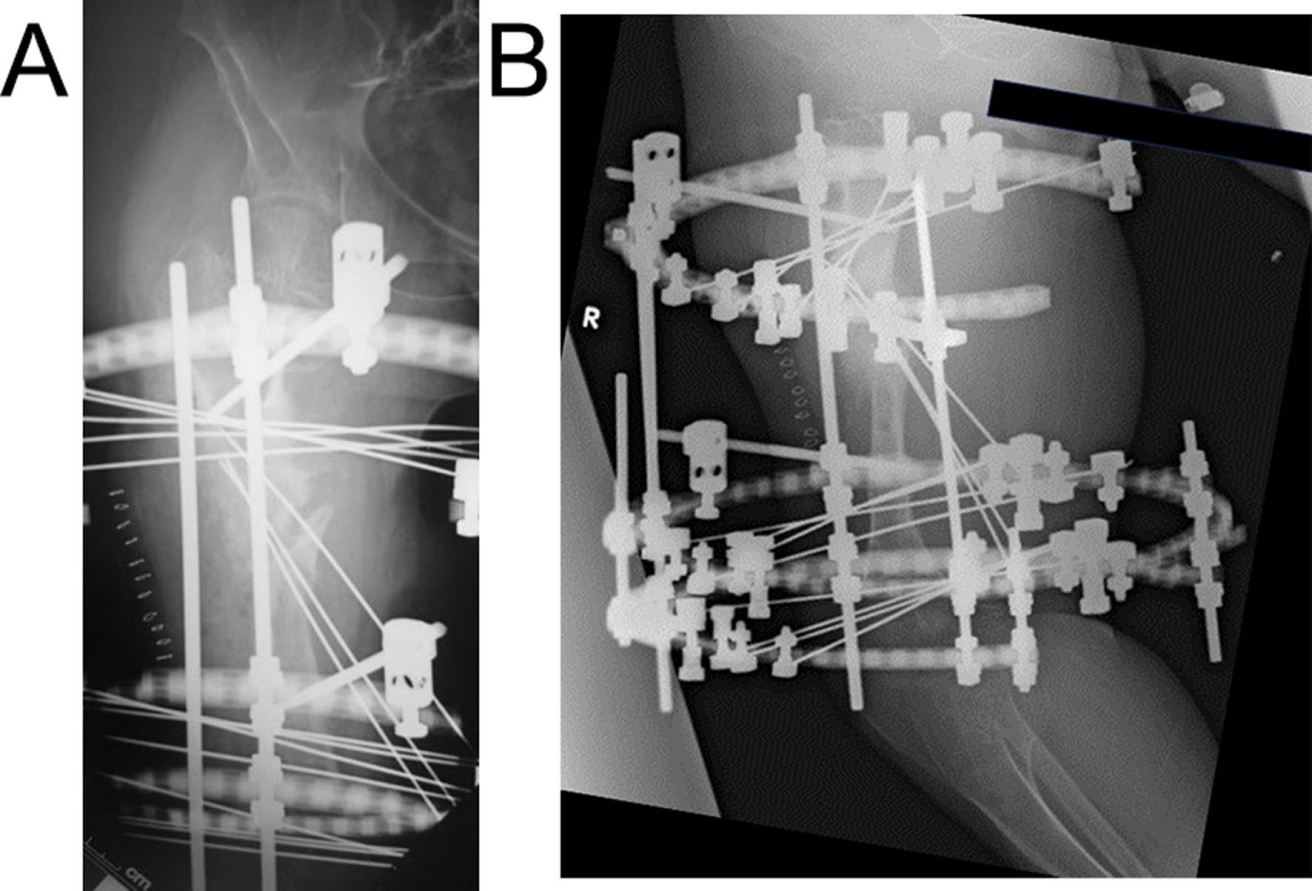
Third surgery postoperative X-rays
